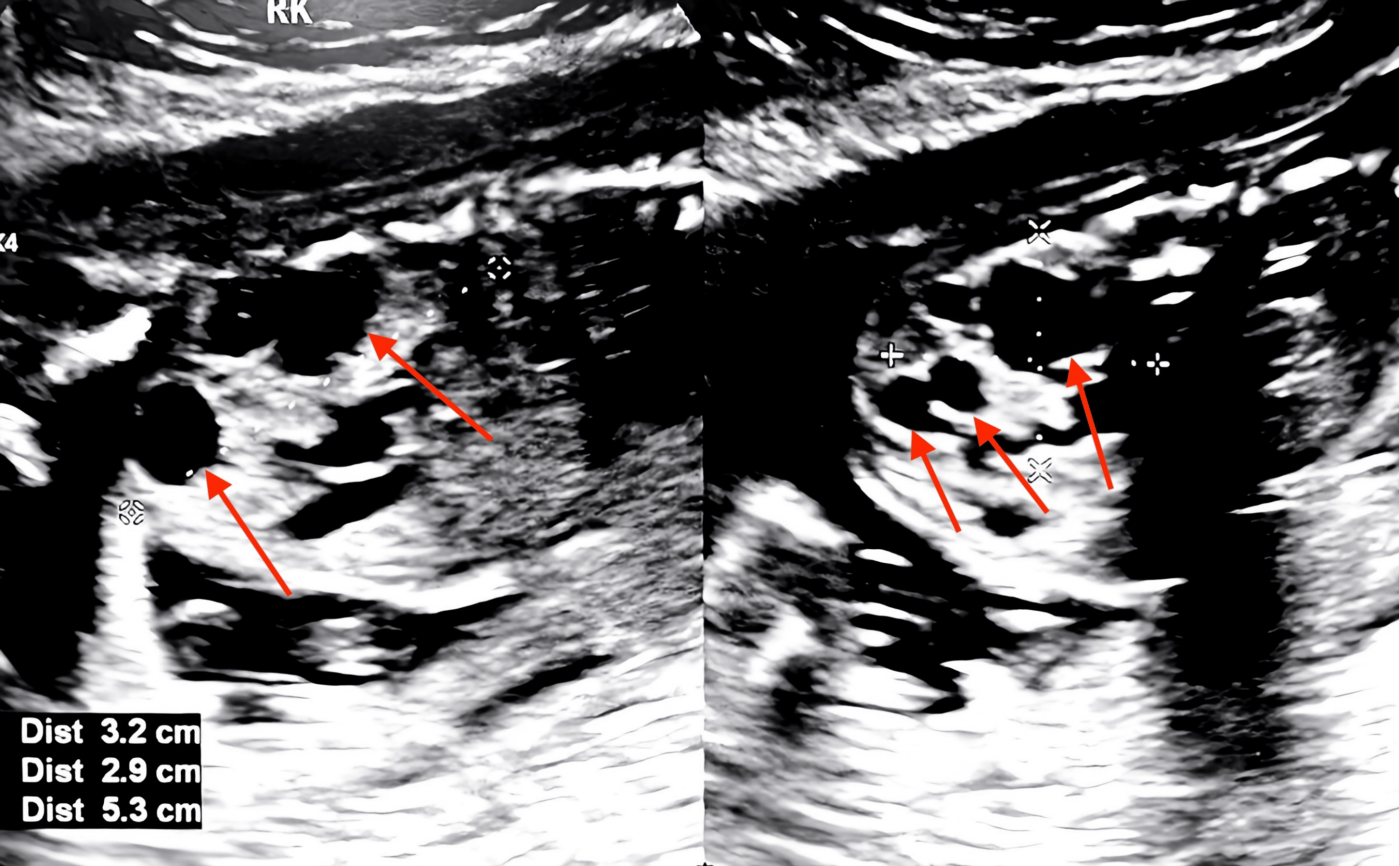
Fetal ultrasound scan at 30 weeks and five days of gestation showing the right multicystic kidney
